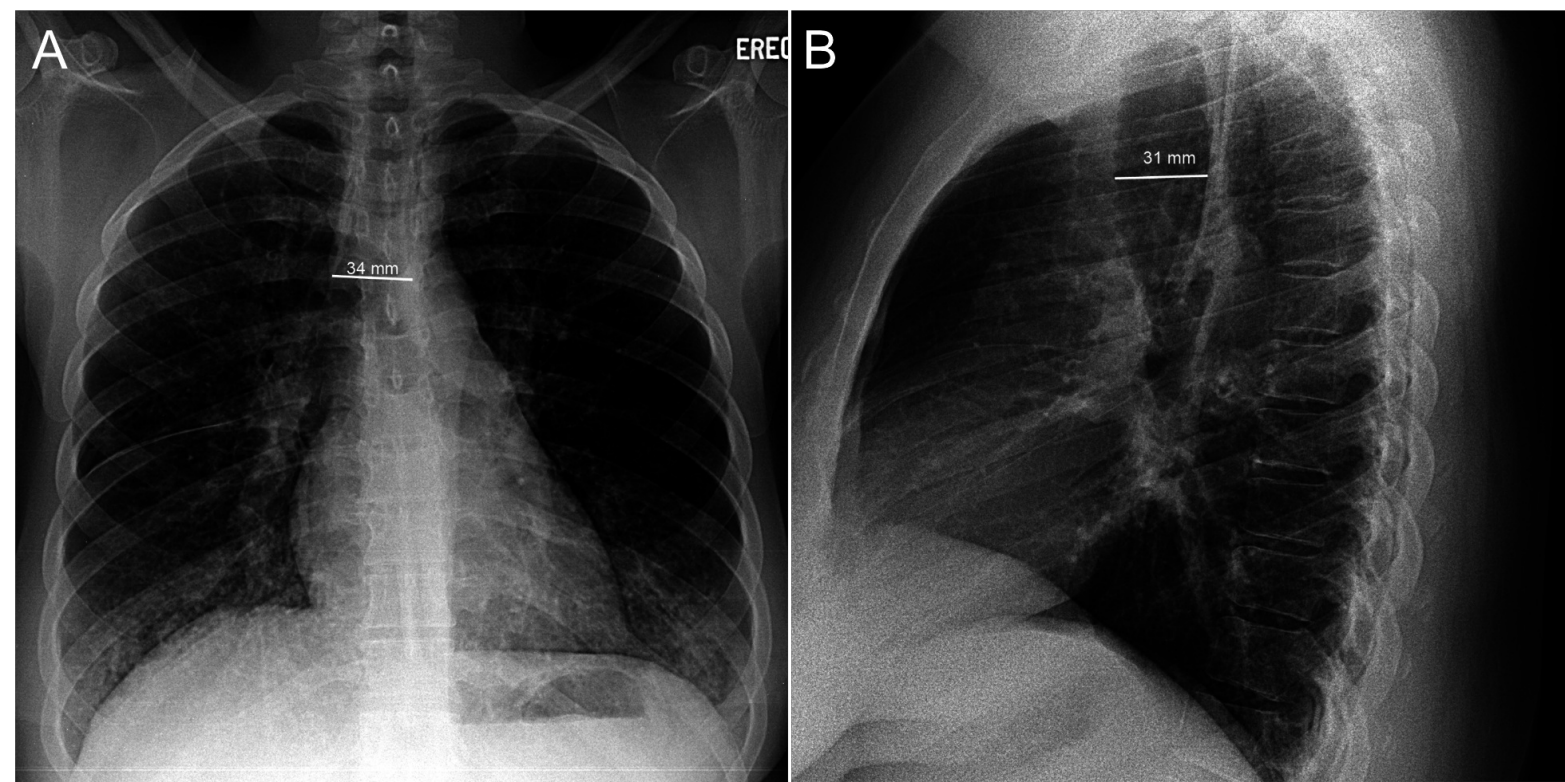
Posterior-anterior (A) and lateral (B) chest radiographs Chest radiographs from fourteen years prior demonstrate unchanged, chronic tracheobronchomegaly.
